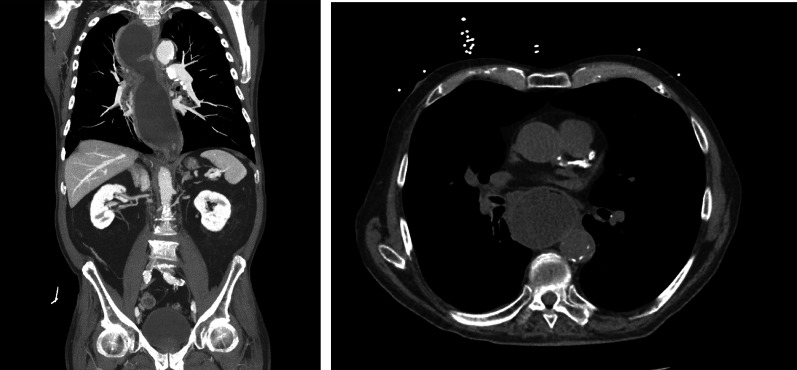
Initial read by the team was concerning for mega-esophagus versus complete herniation of stomach into the chest (stomach was not easily visualized, even on CT). Formal read: diffusely dilated esophagus that can be seen with achalasia. Also plaques within the descending thoracic aorta. No evidence of dissection
